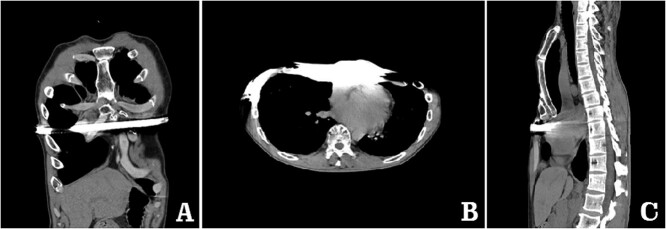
Computed tomography examination before operation. (A) Coronal view; (B) sectional view; and (C) sagittal view.
